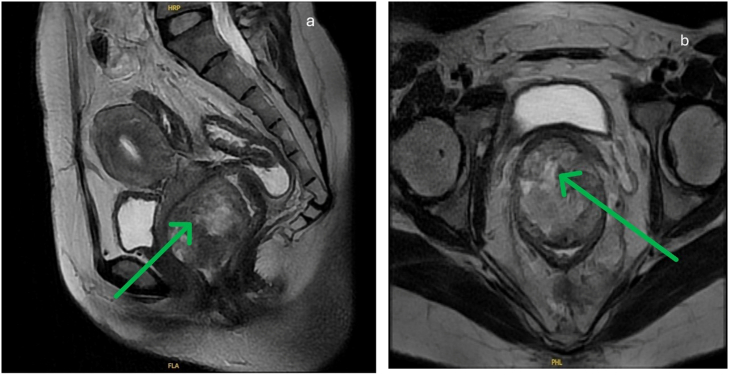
a,b: Pelvic magnetic resonance image showed a 63 mm × 42 mm tumor in the anterior wall of lower rectum (green arrow).
